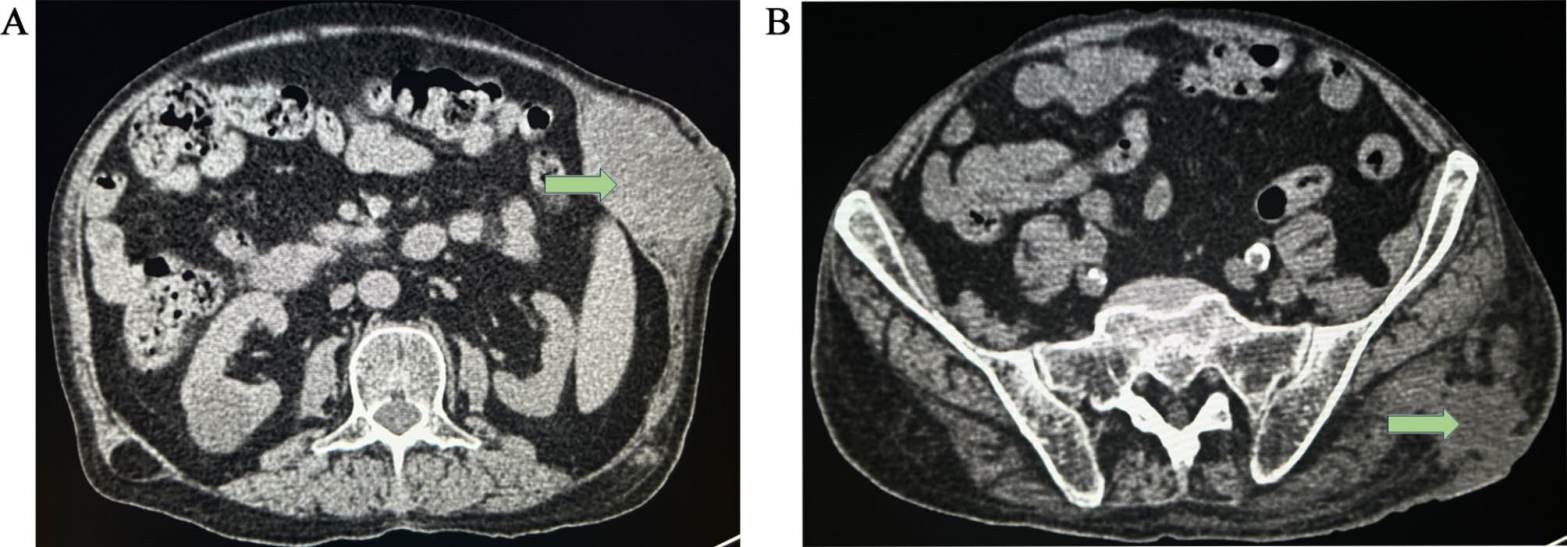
The systematic abdominal CT scan performed showed Soft tissue density mass shadow on the left abdominal wall (A); Subcutaneous watery density mass shadow in the left buttock area (B)(Arrows)
